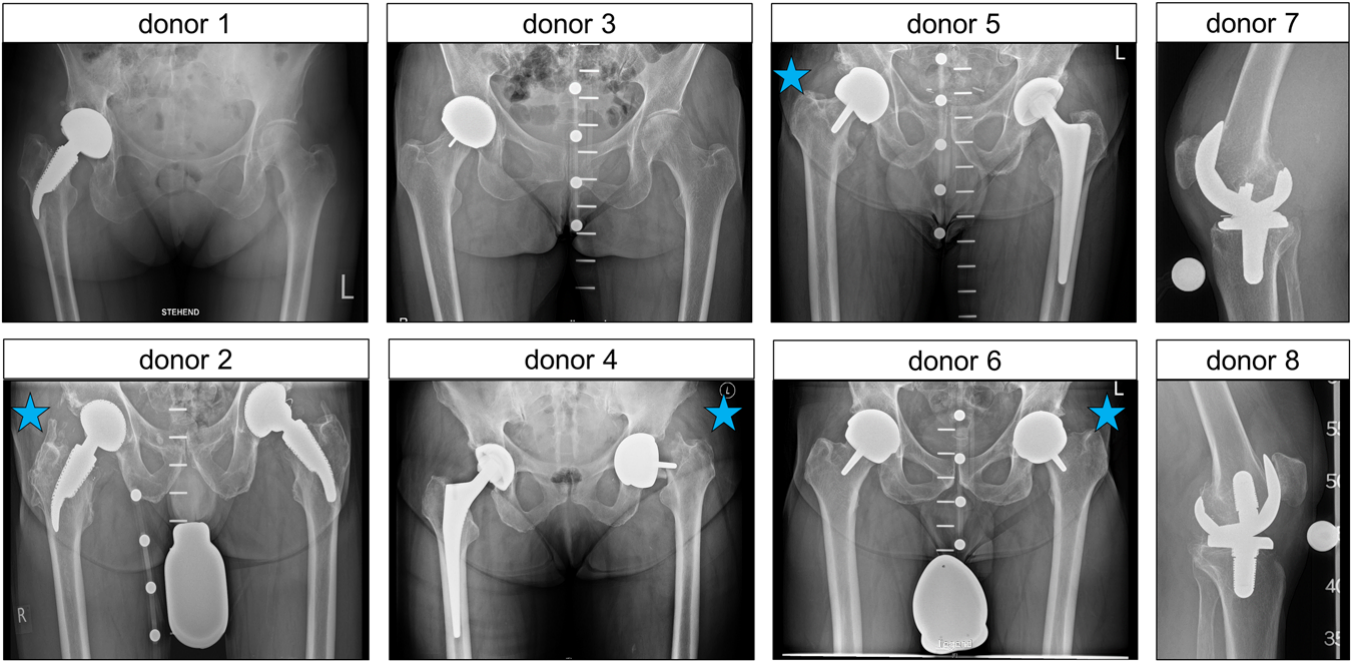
Radiographs taken before index revision surgery, i.e., bone marrow sample extraction (donors 1–6, low-centered ap pelvis; donors 7 & 8, lateral knee view). Blue asterisks indicate the respective index joint. Donor 1–2, modular short stem total hip arthroplasty; donor 3–6, hip resurfacing implant; donor 7–8, total knee arthroplasty
